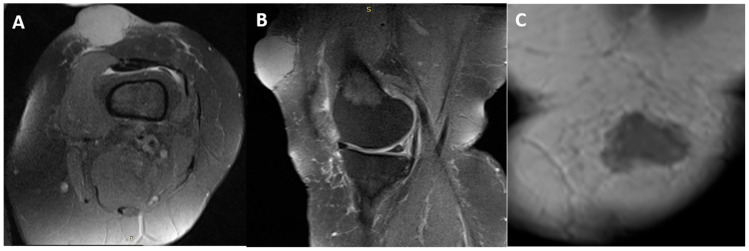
T2 post-contrast magnetic resonance imaging of the left distal thigh demonstrating an extracompartmental subcutaneous mass without quadriceps muscle invasion (representative (A) axial, (B) sagittal and (C) coronal images).
